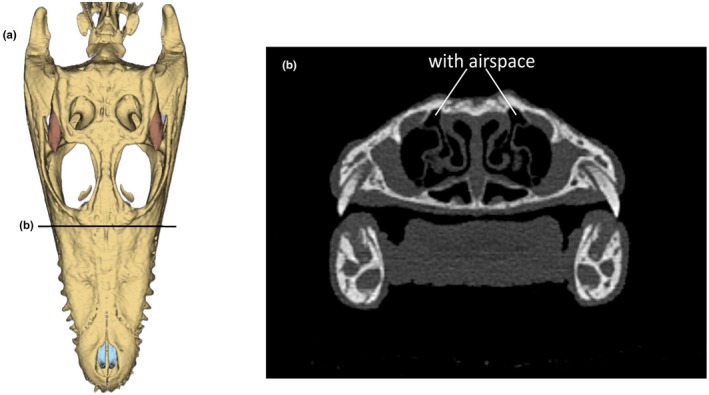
(a) Skull rendering of a juvenile *Crocodylus rhombifer* (adapted from Melkersson et al., 2024) and (b) CT slice showing the olfactory region of *Crocodylus rhombifer*.
